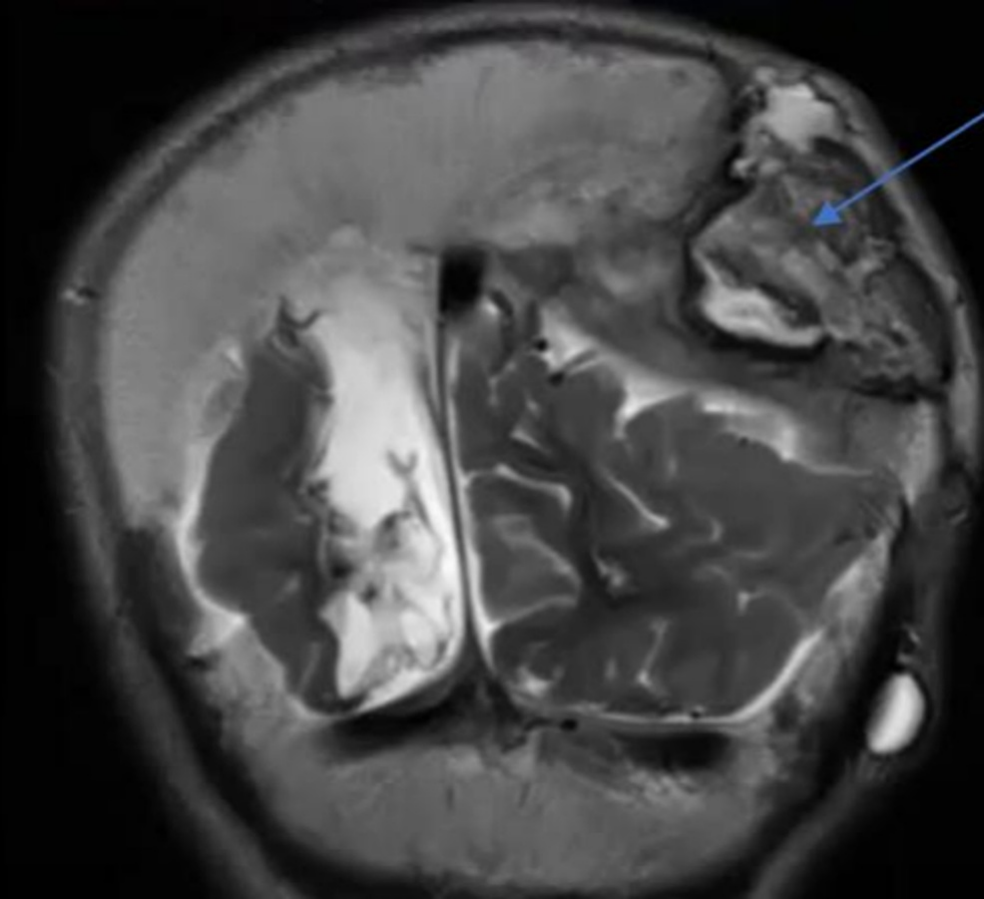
An MRI brain scan showed an enhancing epidural collection of 4 cm thickness (pointed in the image).
